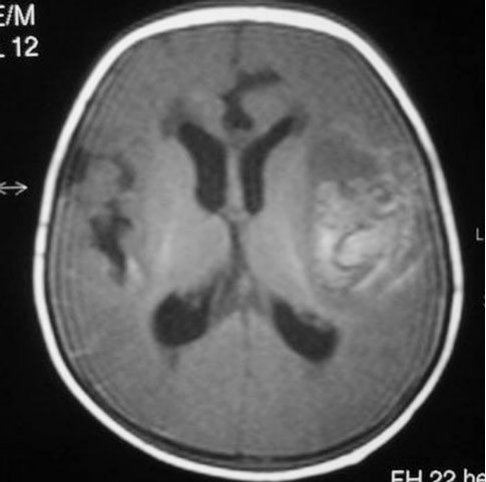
Axial T_1_-weighted cranial MR image shows hemorrhages in temporal lobe
